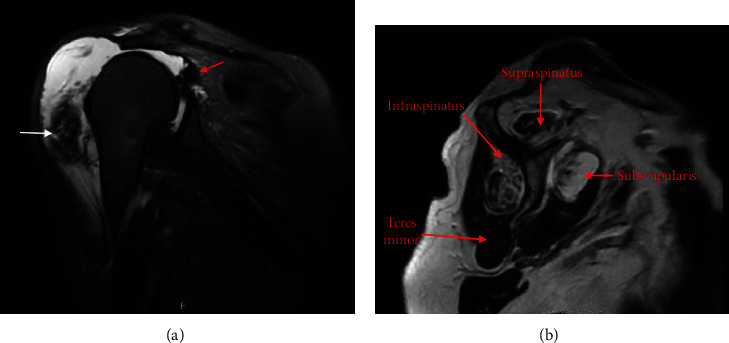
Coronal (a) and sagittal (b) view of a T2-weighted MRI of the right shoulder showing a large effusion with rupture of the deltoid (white arrow) and rotator cuff tear (red arrow).
